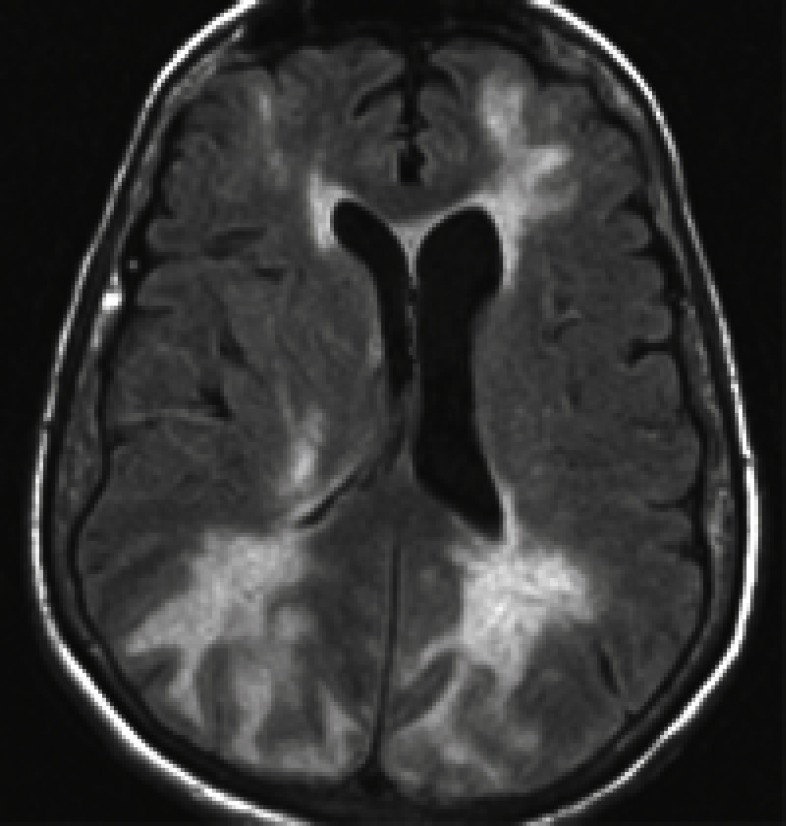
Brain MRI in a patient with posterior reversible encephalopathy syndrome (PRES) shows multiple areas of hyperintense signal
